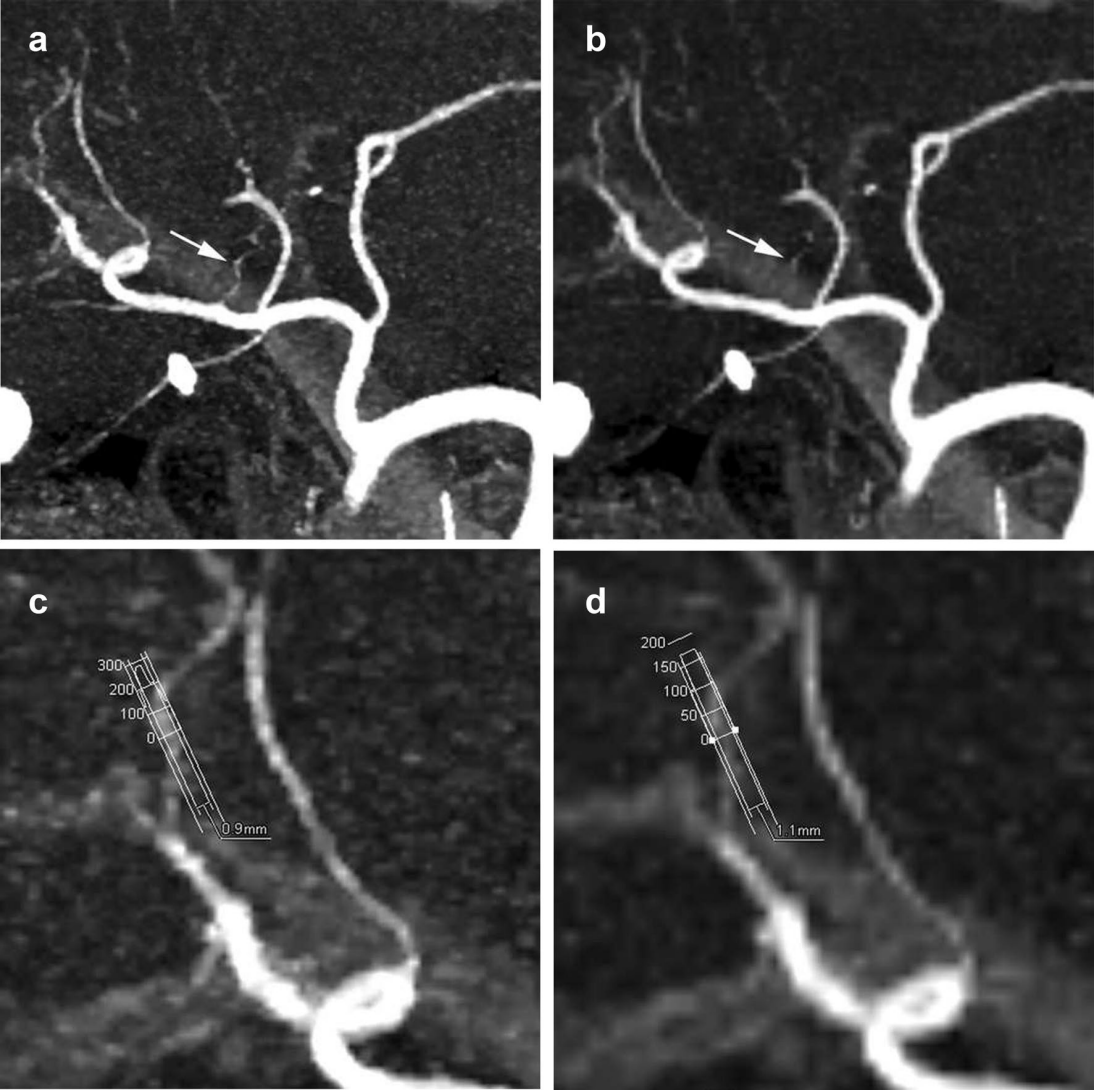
Partial MIP image of hepatic CTA. a: HR CTA with a matrix of 1024 × 1024 using the UHR CT scanner more clearly showed a small branch (A1) of the hepatic artery (arrow) than (b): NR CTA with a matrix of 512 × 512. Profile curves of the small hepatic artery of segment VIII of (c): HR CTA and d : NR CTA
